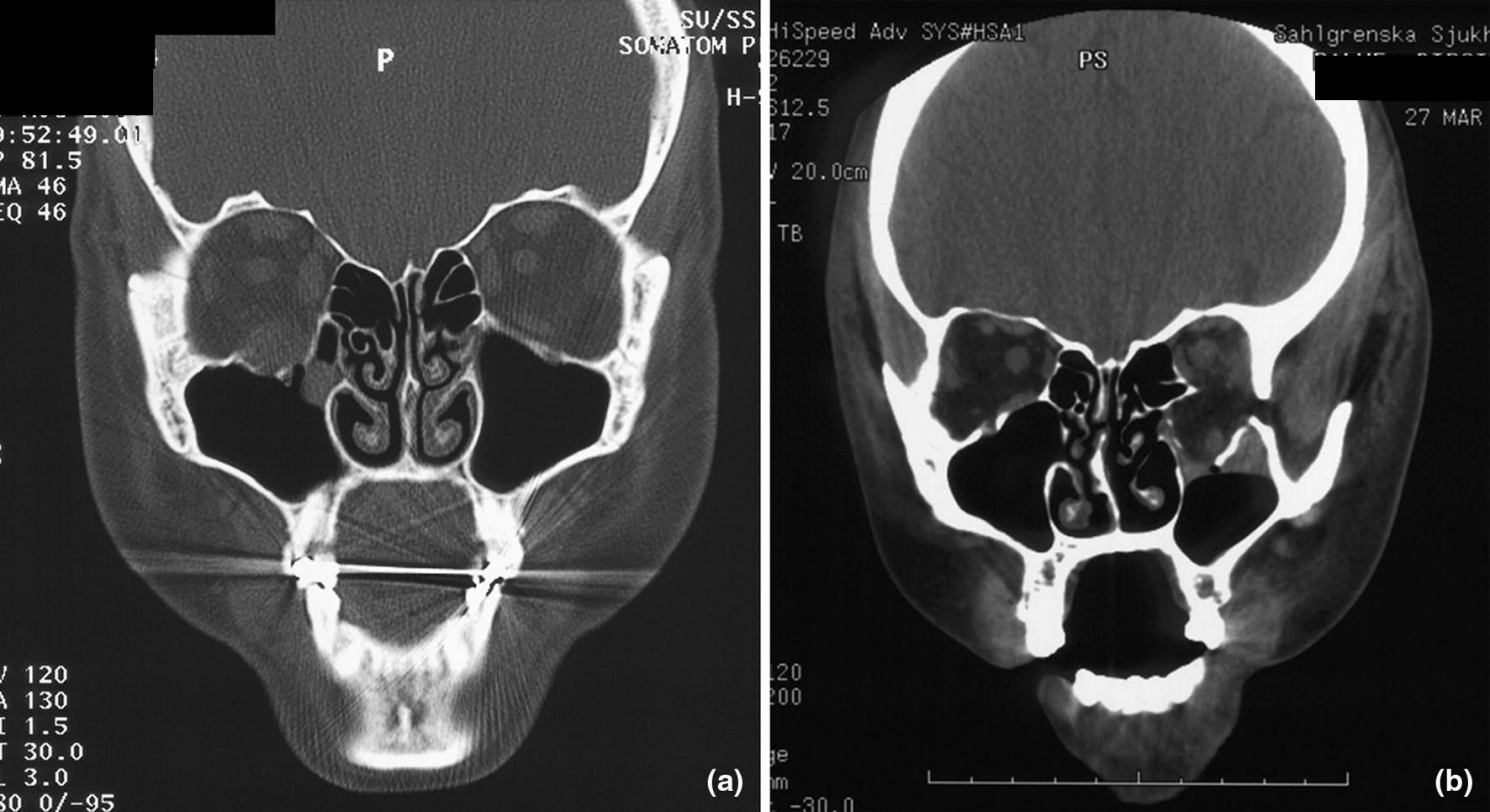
a, b “Rounding” of the inferior rectus muscle in coronal CT scans with an orbital floor fracture at the a right side, b left side, respectively
